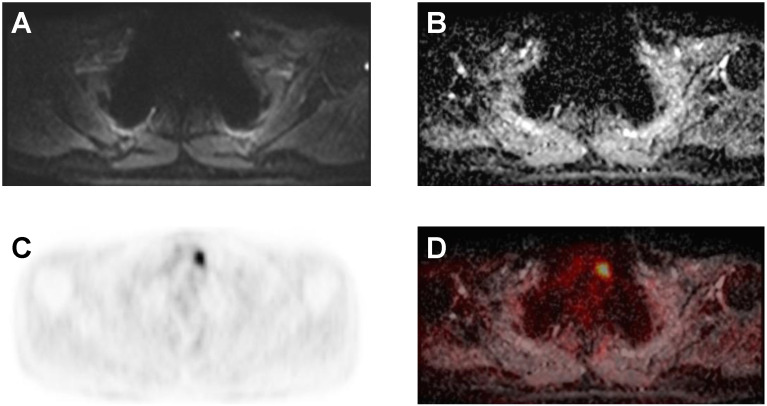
Example of a lesion detected in the right digastric jugular space at MR with inhomogeneous hyperintensity in the T2 sequences (A), restricted diffusivity in the DWI sequences (B), and increased uptake of ^18^F-FDG on PET (C) and PET/MR (D).
